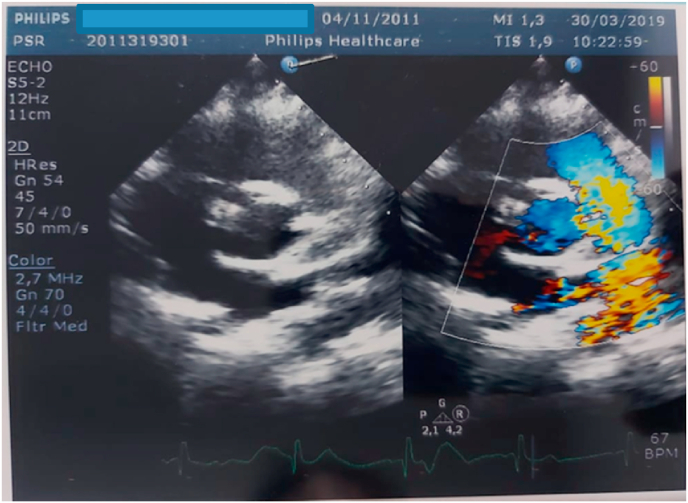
Long Axis Short axis view revealed no residual AP Window from 2D echocardiography and colour Doppler. (For interpretation of the references to colour in this figure legend, the reader is referred to the Web version of this article.)
